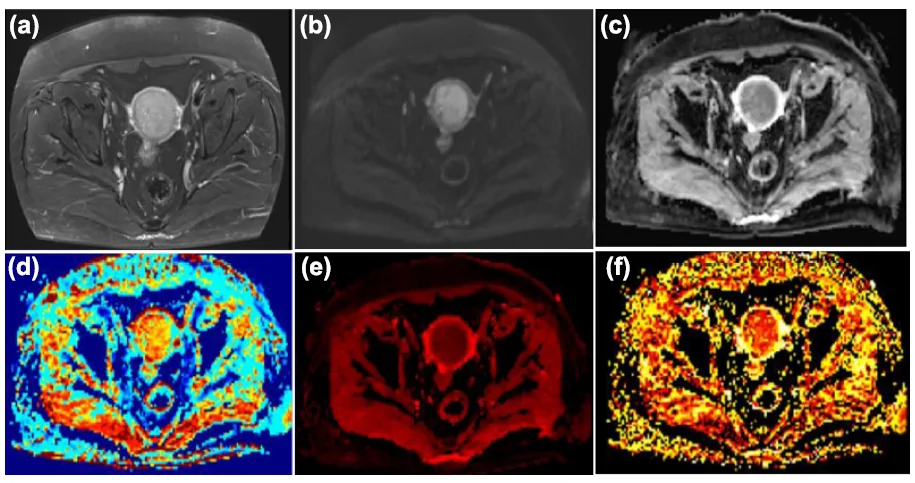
A 68-year-old woman was diagnosed with endometrial carcinoma with the pathological grade of G3. A-C. Transverse T2 weighted imaging (T2WI) in figure (a), diffusion weighted imaging (DWI) in figure (b), and apparent diffusion coefficient (ADC) images in figure (c). D-F. Pseudo color images for the parameters of the fractional order calculus (FROC) model β (d), D (e), and μ (f).
